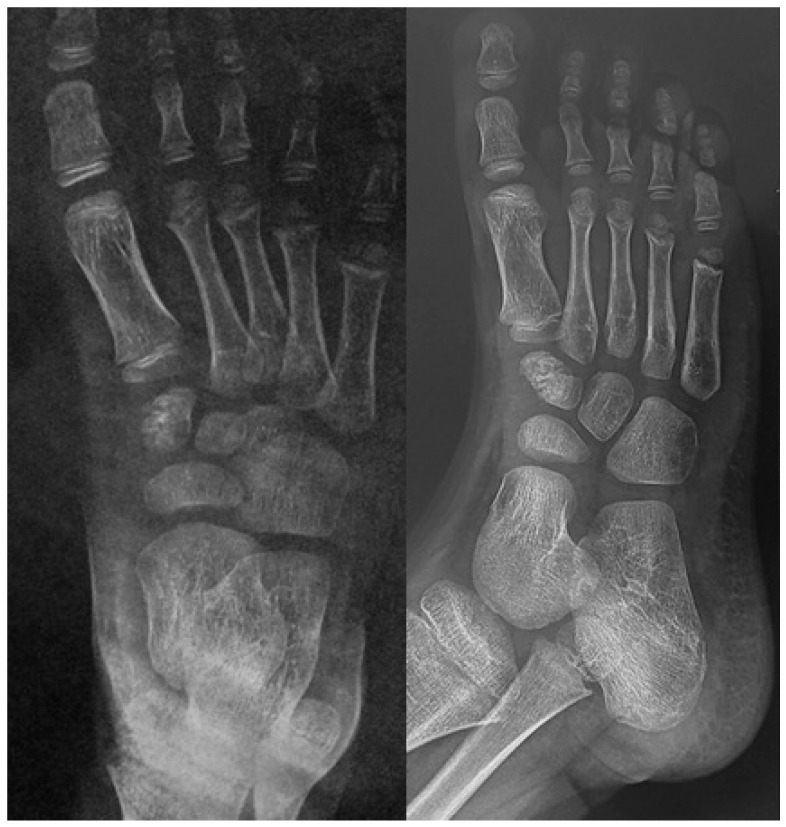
Radiograph of a 9-year-old male patient demonstrating fragmentation and irregular margins of the medial cuneiform bone.
